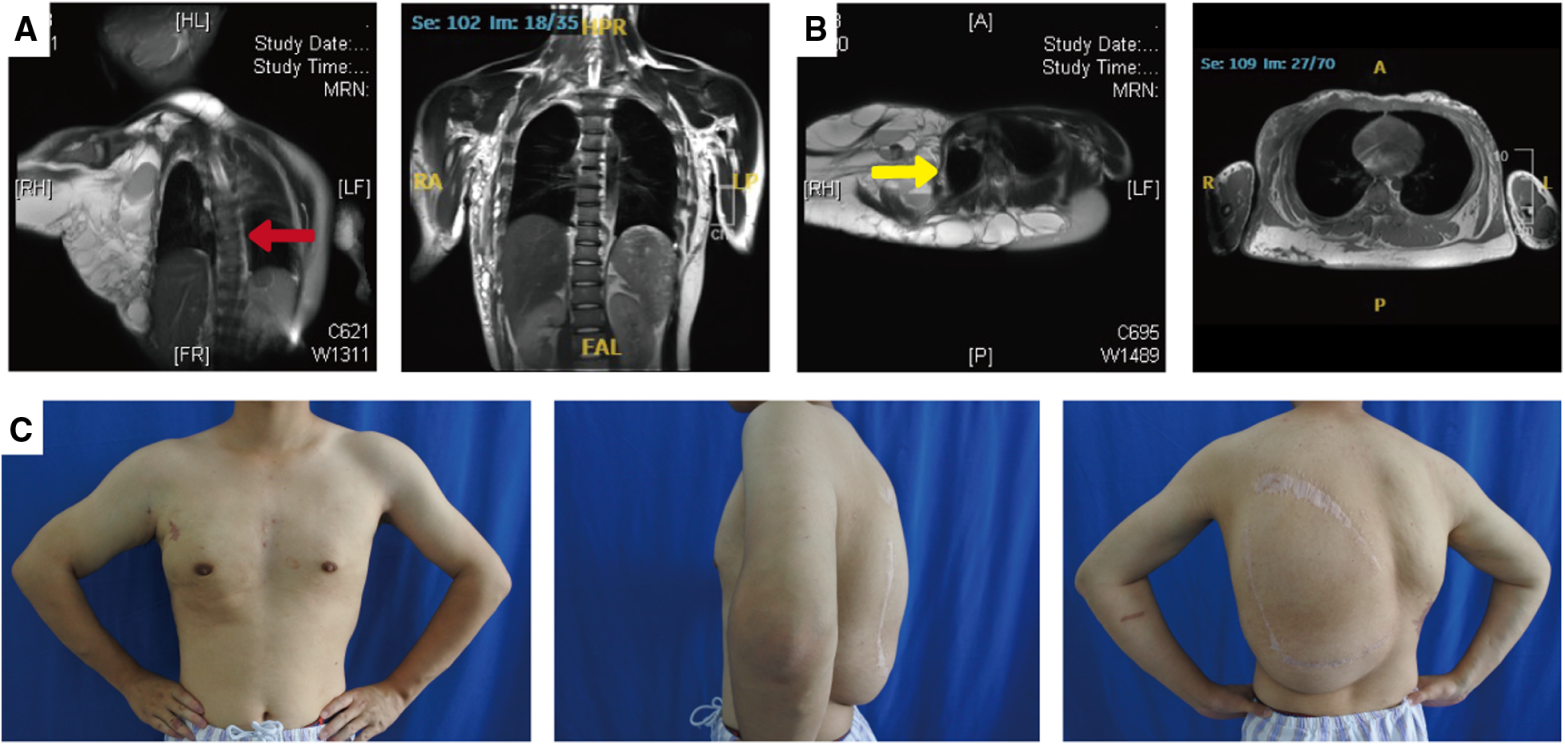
Photographs and MRI images of the patient's spine and thorax before and after treatment. Magnetic resonance imaging showed scoliosis (A; red arrow) and thoracic compression deformity (B; yellow arrow). After treatment, the patient has a normal spine (A, right panel) and a symmetrical thorax (B, right panel). The patient's arms and chest have a nearly normal appearance, and the back mass has subsided significantly (C).
